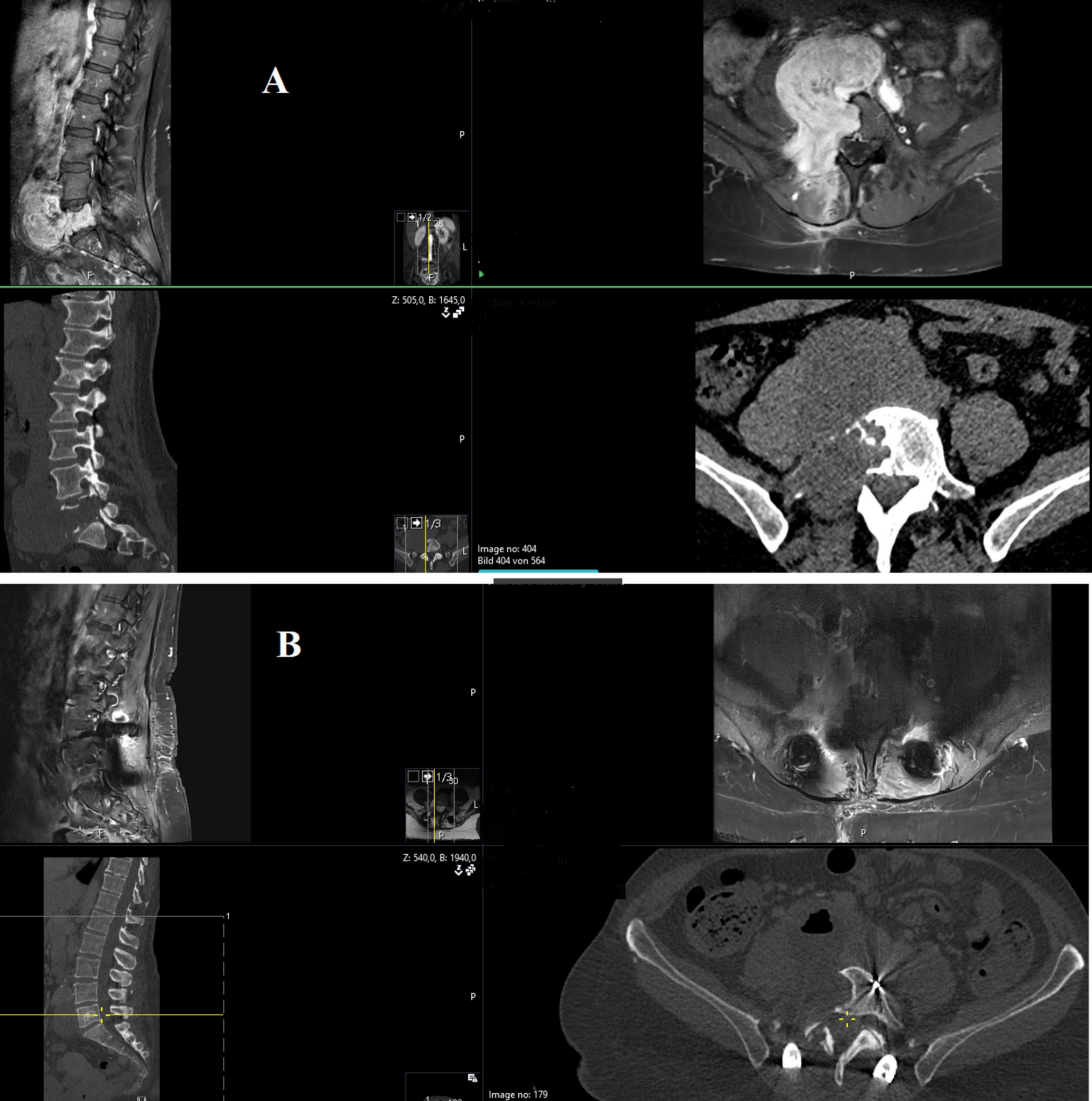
A Preoperative contrast-enhanced MRI and CT imaging revealed a large, heterogeneously enhancing tumor with extensive bony destruction involving the facet joint, pedicle, and approximately 50% of the L5 vertebral body. B Postoperative contrast-enhanced MRI and CT following right-sided dorsal hemilaminectomy and transpedicular tumor resection to the anterior margin of the L5 vertebral body, with a second-stage transretroperitoneal resection of the remaining tumor. The MRI shows no residual tumor, and the CT confirms correct placement of pedicle screws in L4 and S1. (Carbon Fiber–Reinforced Pedicle Screws)
